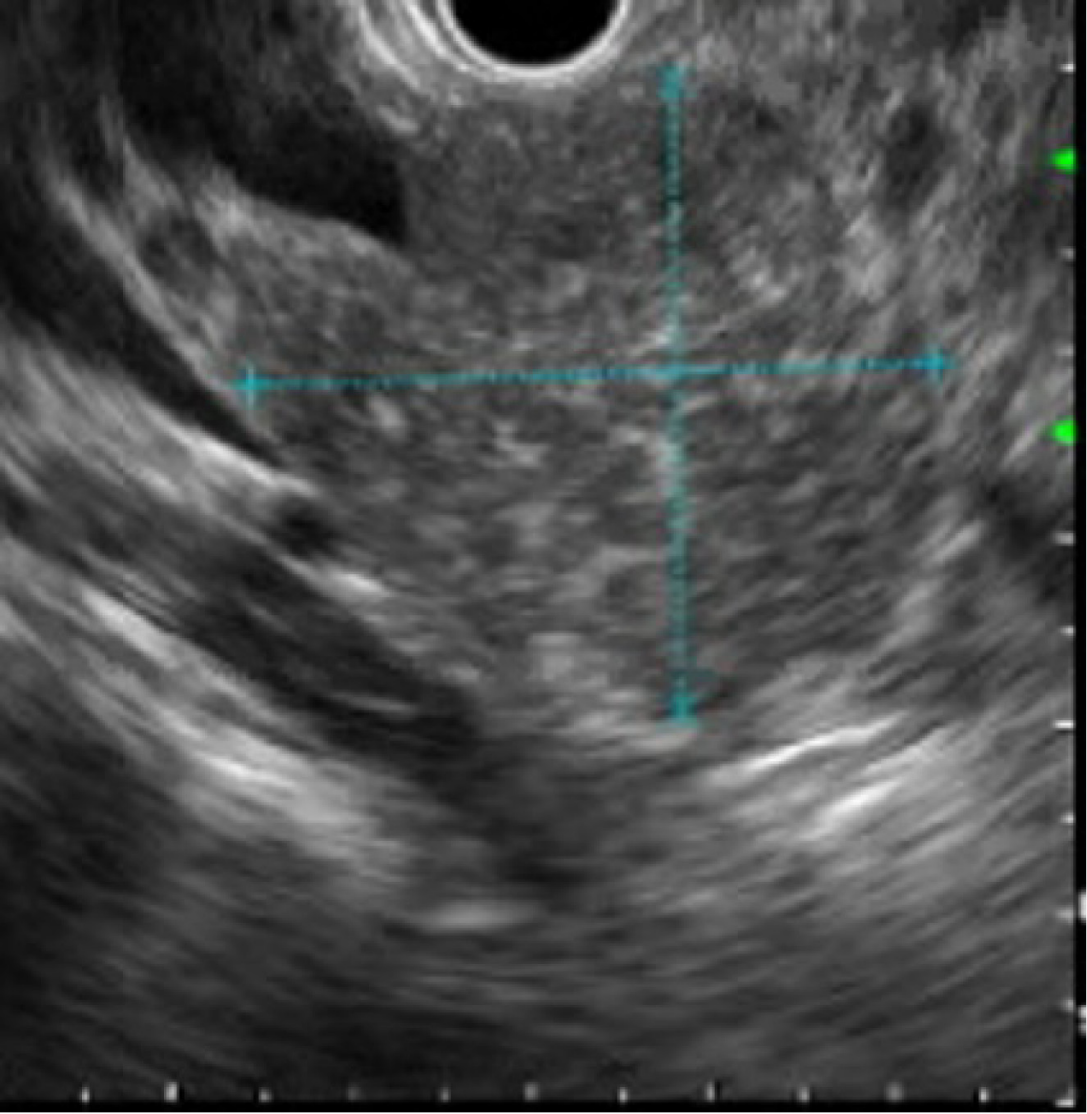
Endoscopic ultrasound findings significant for a heterogenous pancreatic parenchyma without a definite mass.
